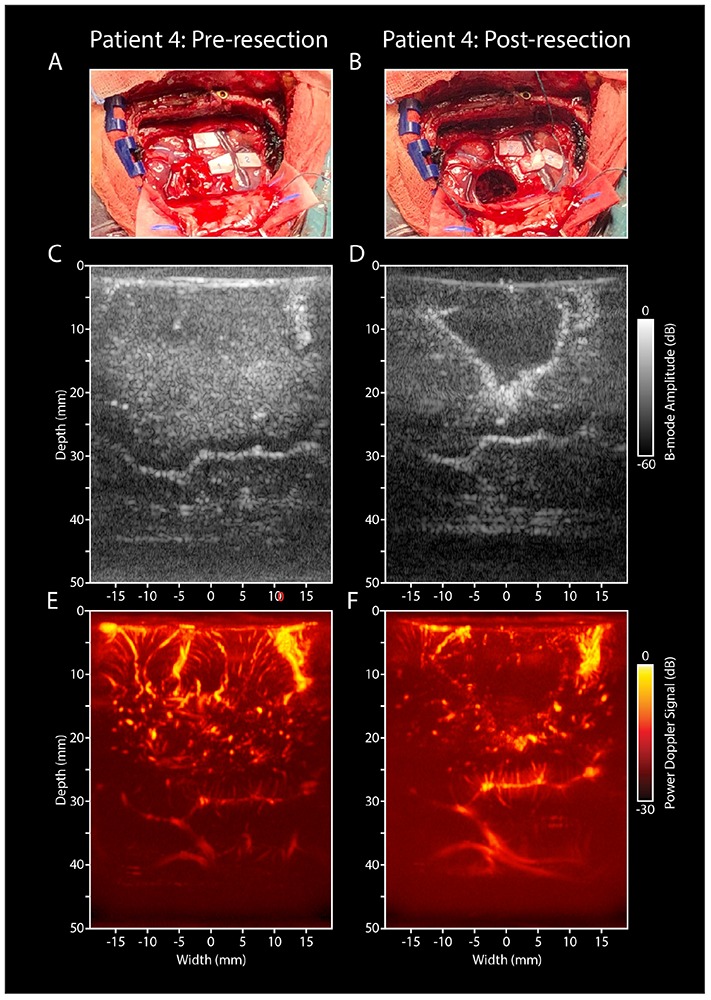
Pre- and post-resection tumor and resection cavity imaging in pt.#4. (A) Intra-operative image of a LGG in the right parietal lobe in pt#4, who presented with several eloquent areas in the proximity of the tumor (see ESM-markers). (B) Intra-operative image of the post-resection cavity after tumor removal. (C) Pre-resection B-mode image of the tumor and surrounding structures. (D) Post-resection B-mode image, showing the hypodense resection cavity created after tumor removal. (E) PDI of the pre-resection field of view covering the tumor and surrounding healthy tissue. (F) PDI of the post-resection field of view depicting the resection cavity, filled with saline. Noticeable are the similar vascular structures depicted in the depth (3–4 cm) of both images (E,F), indicating a similar field of view pre- and post-resection. LGG, low grade glioma; PDI, power doppler image.
